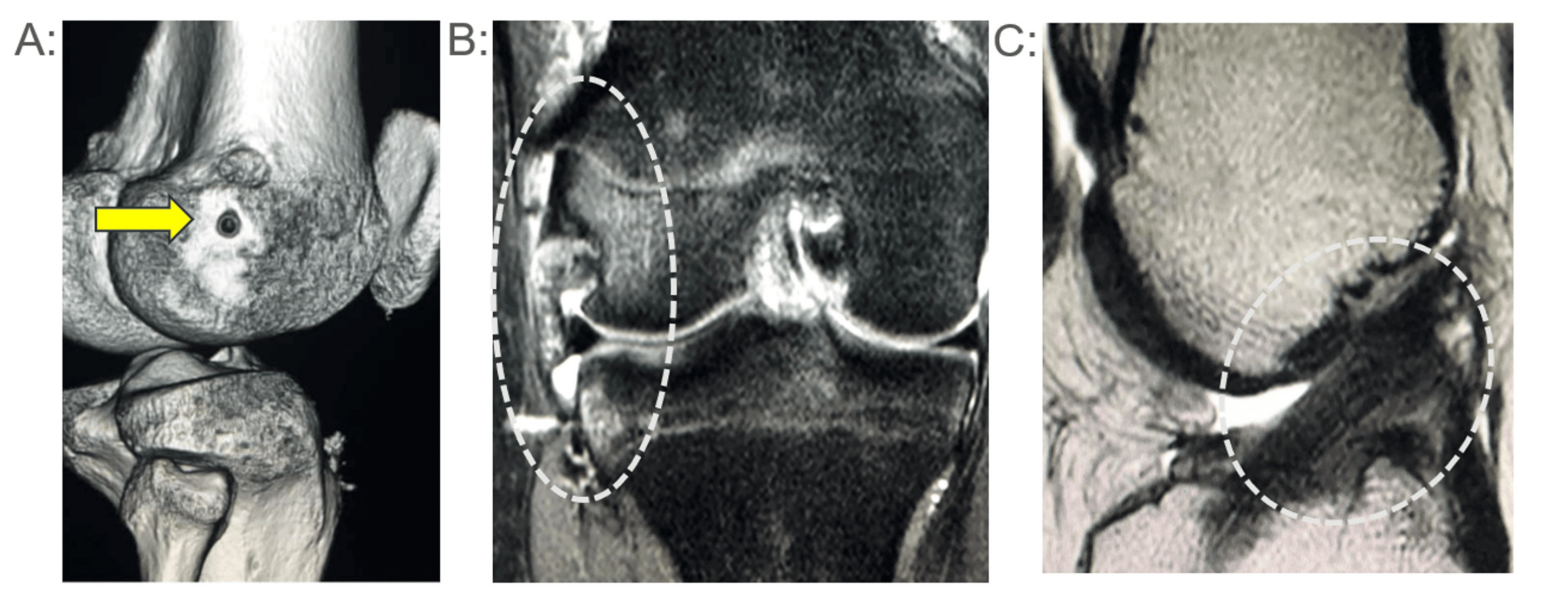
Case 1 (CT・MRI) (A) Postoperative CT scan. The arrow indicates the location of the bone tunnel for the lateral extra-articular tenodesis (LET). (B) MRI at the time of reinjury (fat-suppressed image): injury is observed at the site of the LET. (C) MRI at the time of reinjury (T2 sagittal image): the anterior cruciate ligament (ACL) is intact
